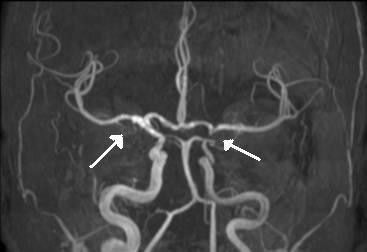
Bilateral carotid vasculitis. Angio-MRI showing straight stenosis of the left carotid artery and inflammatory aspect of the right carotid artery (white arrows).
